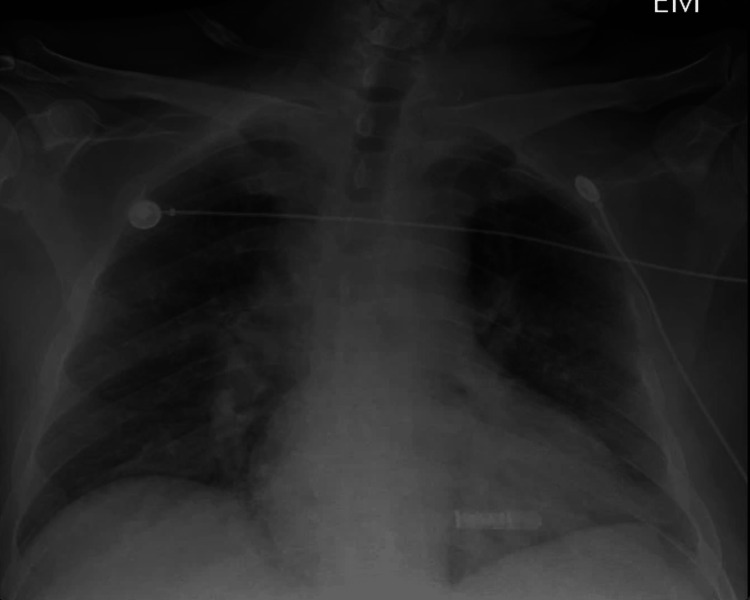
Chest X-ray showing diffuse interstitial pulmonary edema
